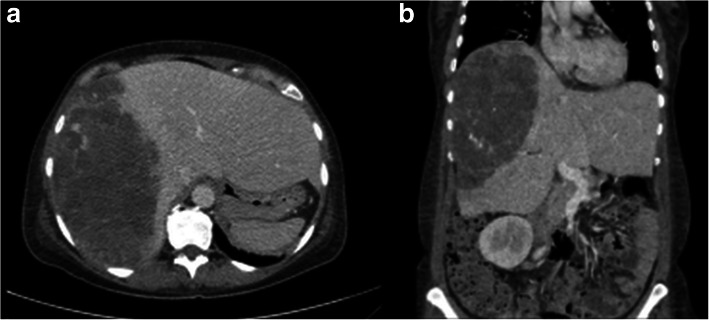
Preoperative contrast enhanced computed tomography scan showed a heterogenous mass in the right liver lobe (a and b)
